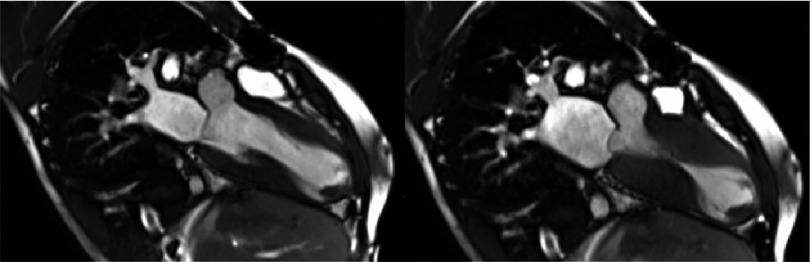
Two chamber view of the heart on cardiac MRI during diastole and systole. There is mid-cavity obliteration during systole; an apical aneurysm with an apical thrombus.
